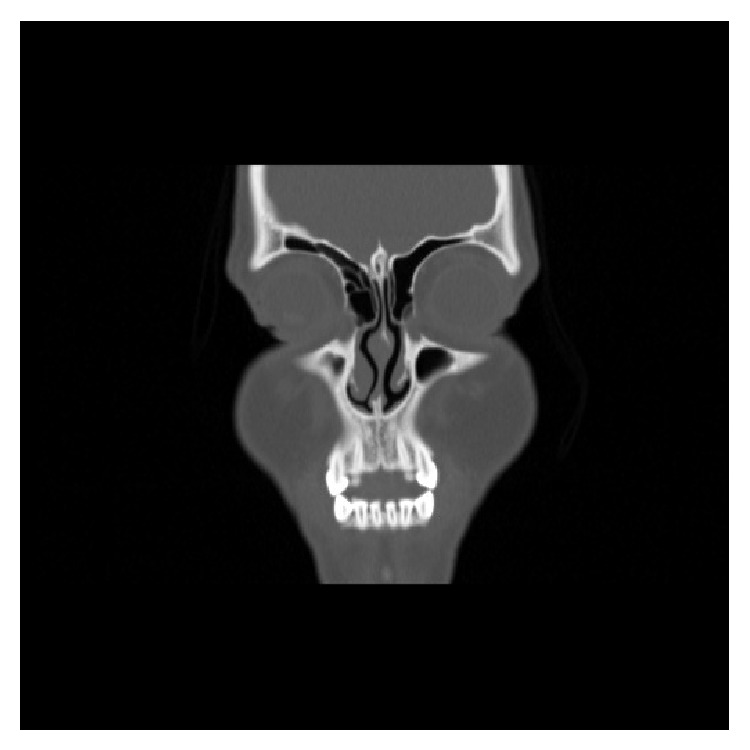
Coronal computed tomography section showing bilateral superior pneumatization.
